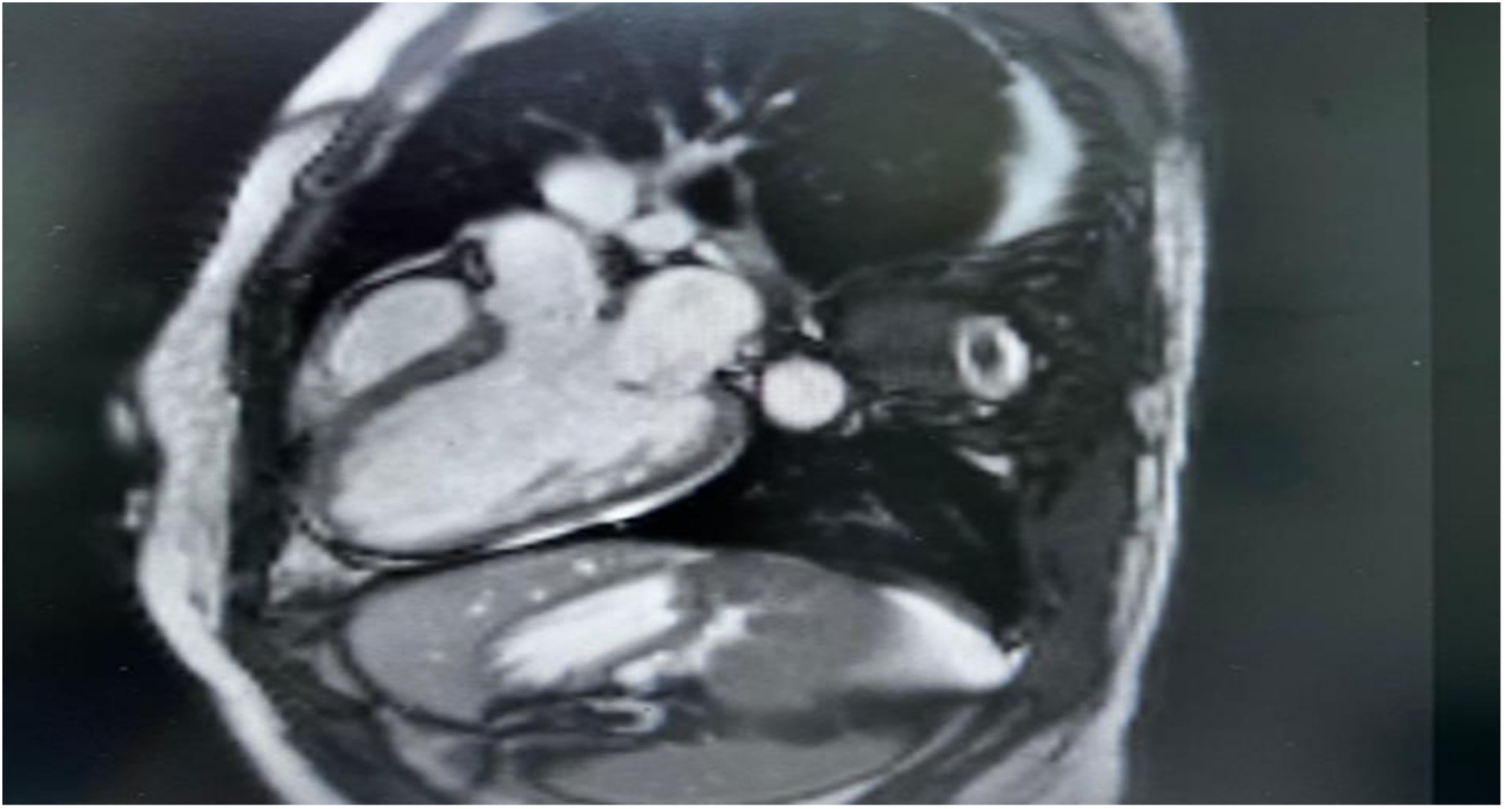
Cardiac MRI, there is non-territorial focal transmural delayed enhancement involving the anteroseptal wall of the mid myocardium. Also there is focal sub endocardial enhancement of the inferior-lateral wall of the mid myocardium. Small right-sided pleural effusion is noted.
